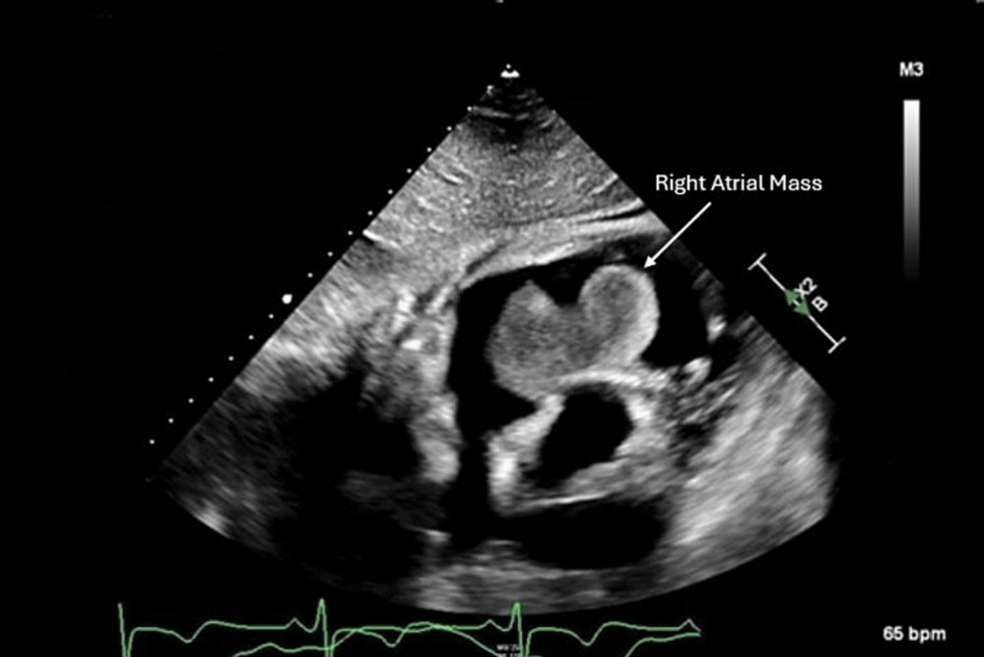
Echocardiography showing a pedunculated right atrial mass
